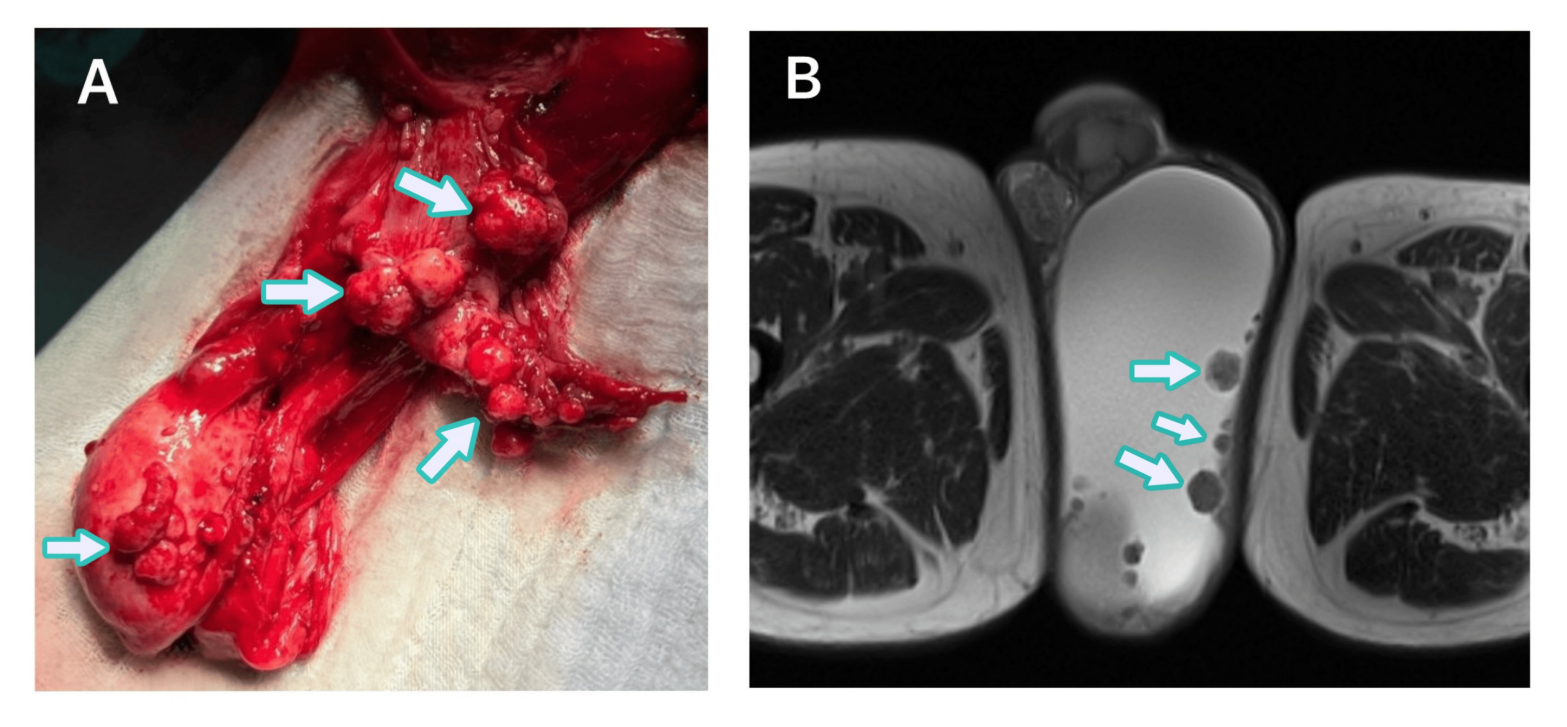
Scrotal exploration and scrotal magnetic resonance imaging (MRI). (A) Scrotal exploration revealed solid tumoral lesions arising from the surface of the testis and spermatic cord. Arrows show tumoral solid lesions. (B) Scrotal MRI revealed solid tumoral lesions located on the tunica vaginalis, in association with a hydrocele and the adjacent testis. Arrows show tumoral solid lesions.
